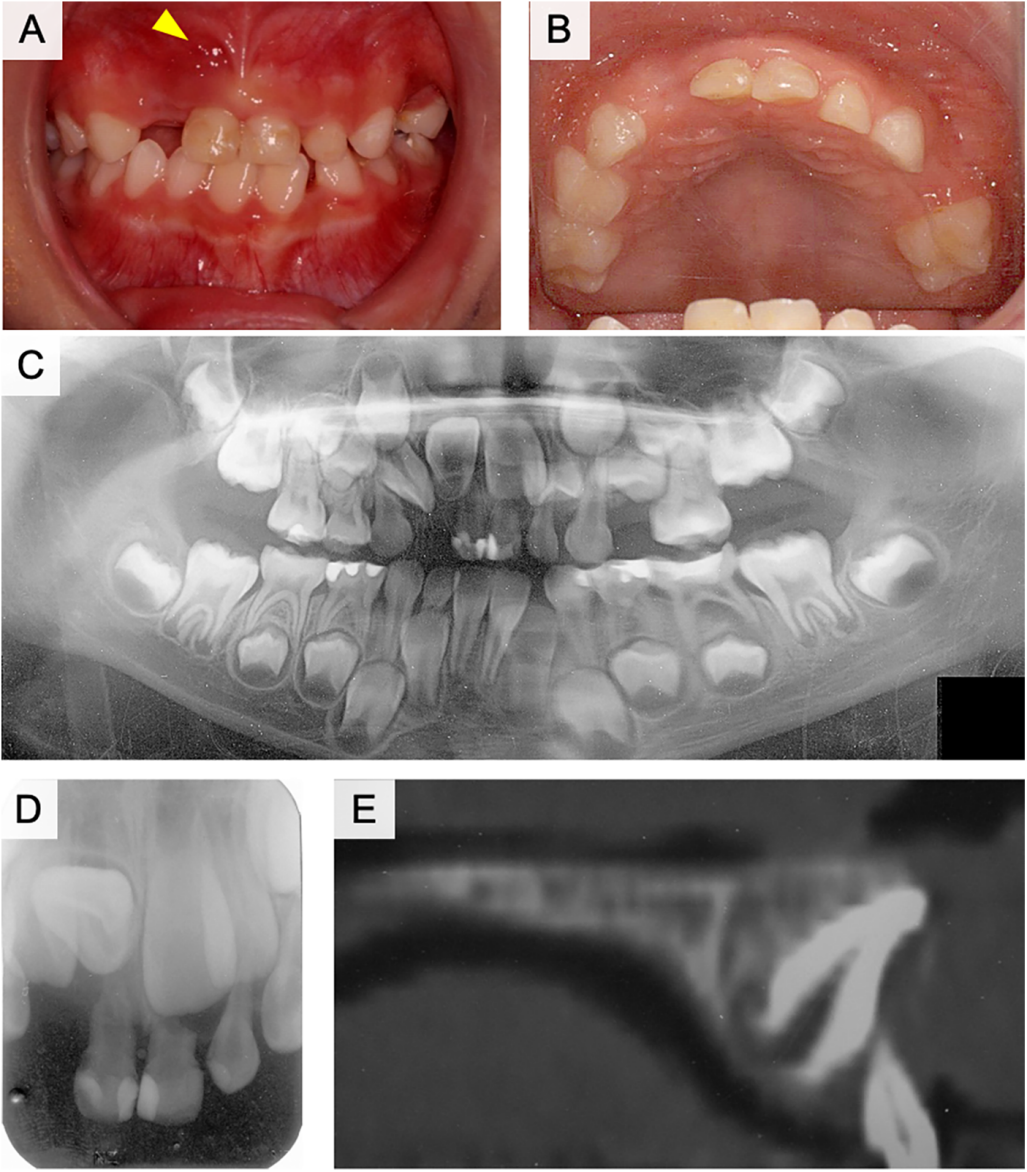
Intraoral and radiographic photographs at the initial examination of case 3. (A) Frontal view of intraoral photograph. (B) Maxillary occlusal view of intraoral photograph. (C) Panoramic radiograph. (D) Dental x-ray image of the right maxillary central incisor. (E) Sagittal plane image of the right maxillary central incisor using computed tomography (CT).
